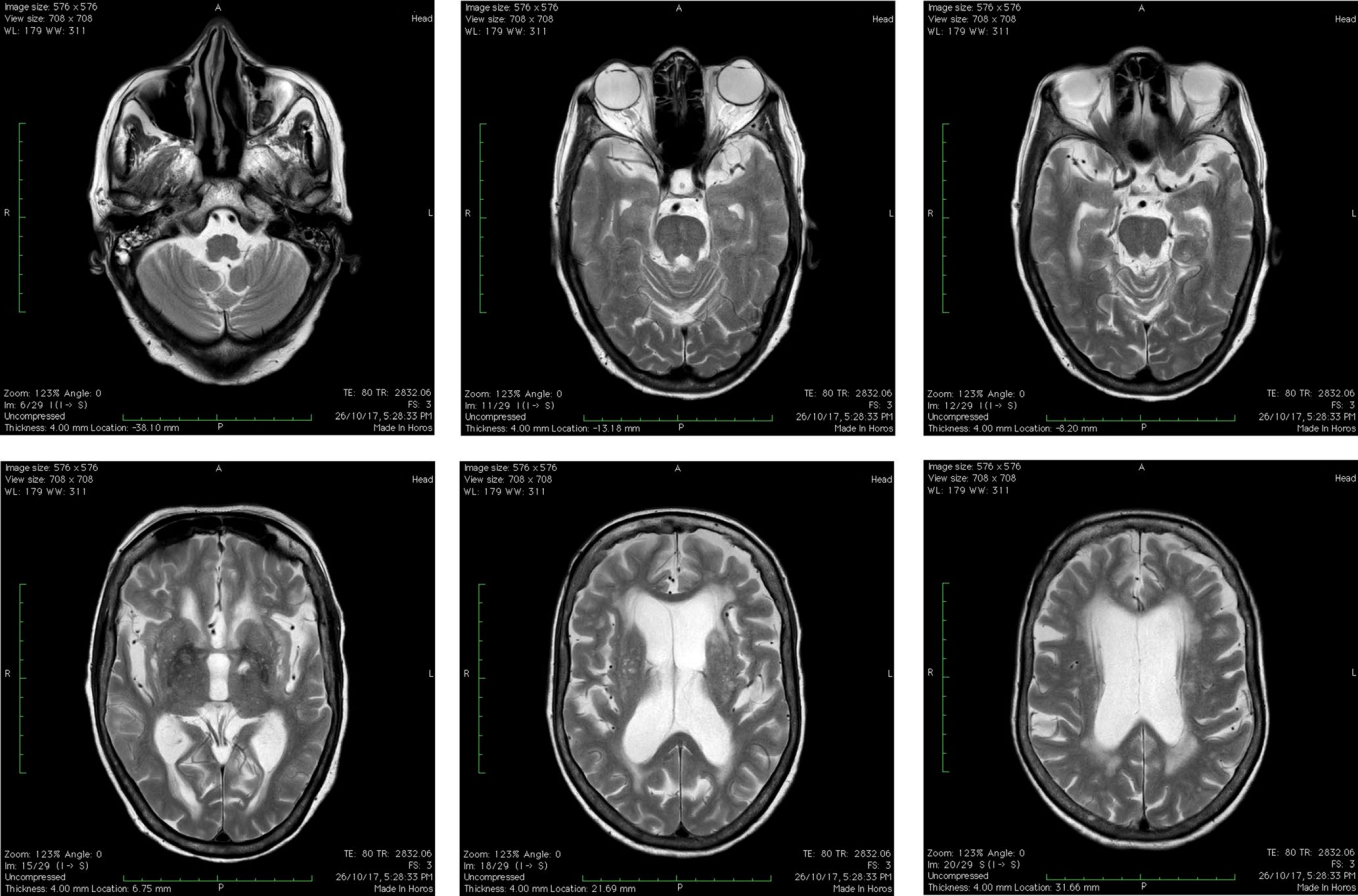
Magnetic resonance imaging of the patient's brain showing normal age‐related changes only
